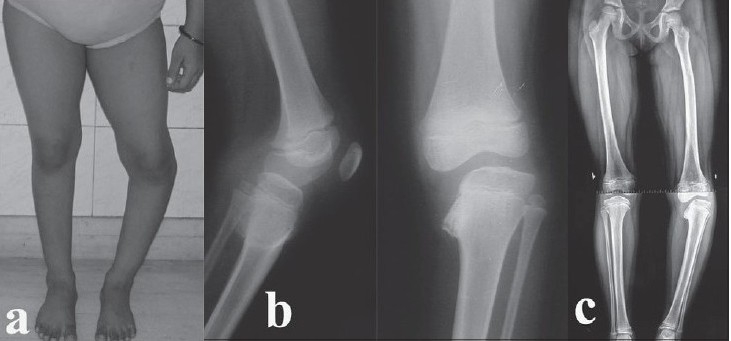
(a) Preoperative clinical photograph of an eight-year-old girl showing a varus deformity of the left proximal tibia. (b) Preoperative lateral and anteroposterior radiographs showing medial beaking and a significant depression of the medial tibial epiphysis and metaphysis (Langenskiold Stage IV). (c) Preoperative alignment-view radiograph of both lower limbs showing a medial mechanical axis deviation and demonstrating the extent of the deformity
